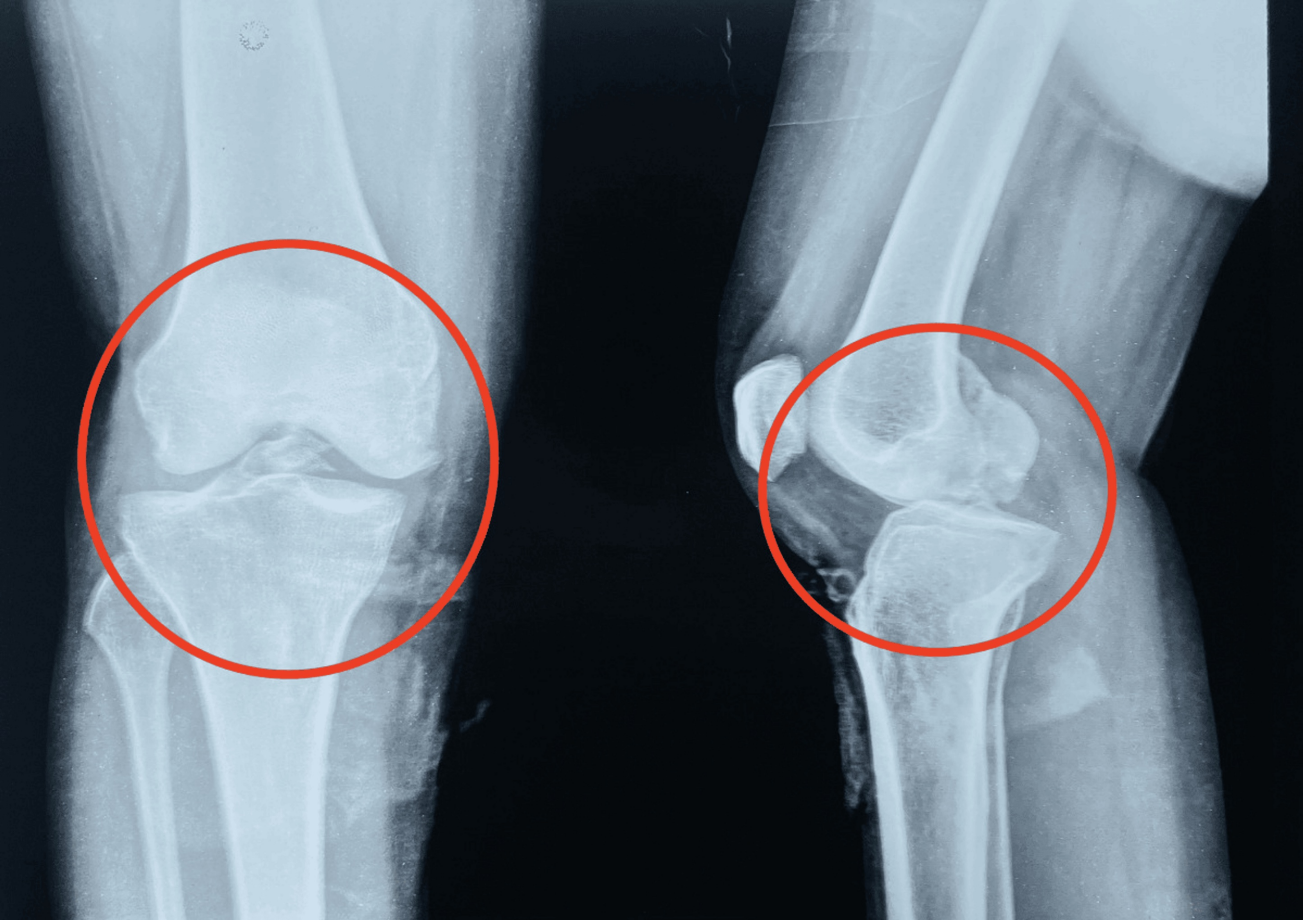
X-ray of the right knee in AP and lateral view showing a tibial condyle avulsion fracture
